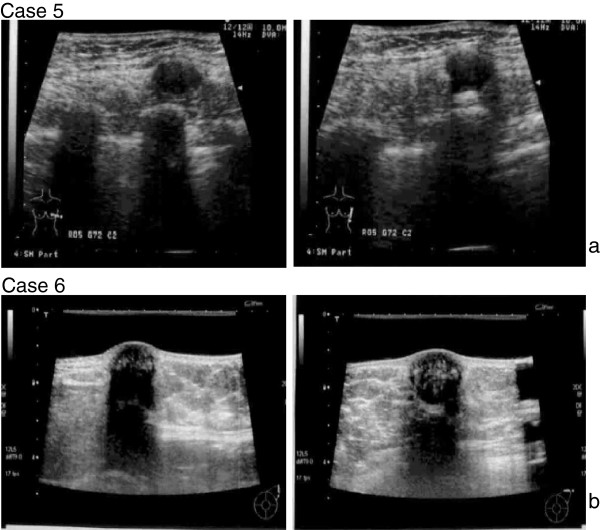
(a) Case 5. Ultrasonography revealed an irregularly bordered, low echoic tumor that protruded in a convex shape from the intrinsic muscle layer of the latissimus dorsi muscle. The size was 10 × 14 mm. (b) Case 6. Ultrasonography revealed a smoothly bordered tumor with posterior echo attenuation, having heterogeneous content and 18 × 17 mm in size.
